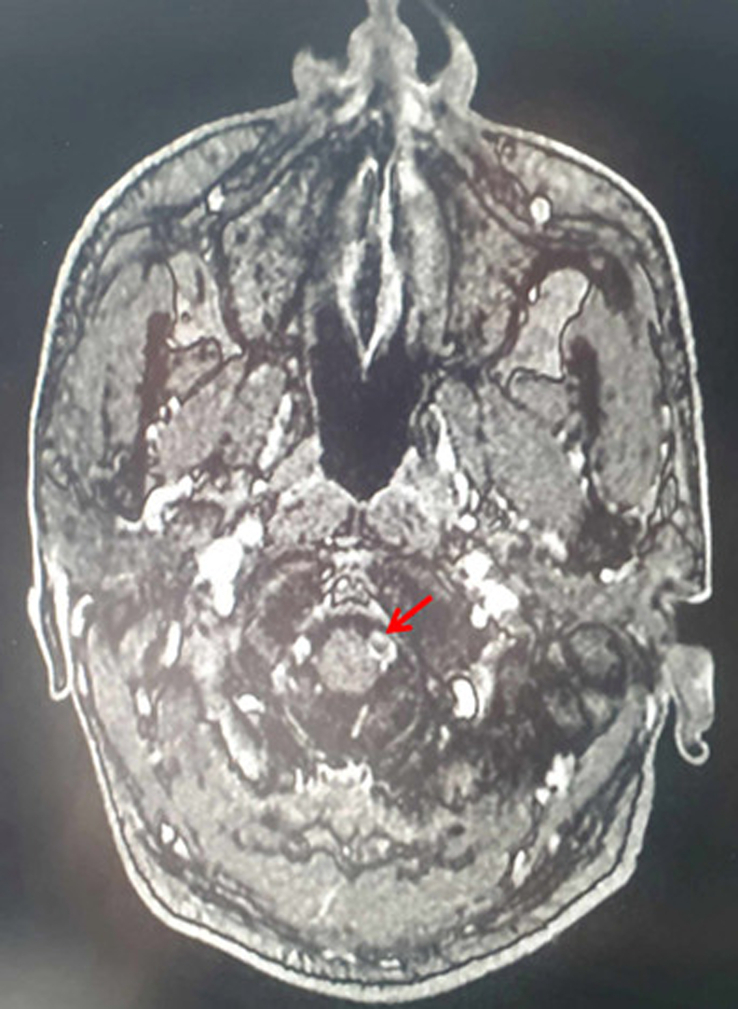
Obliterated left vertebral artery (red arrow) in MRI angiography sequence.
